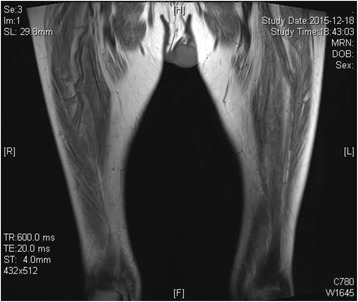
The low extremity MRI. Mild atrophy of both thigh muscle with uniformly increased signal intensity, which is accordant with the features of myopathy
